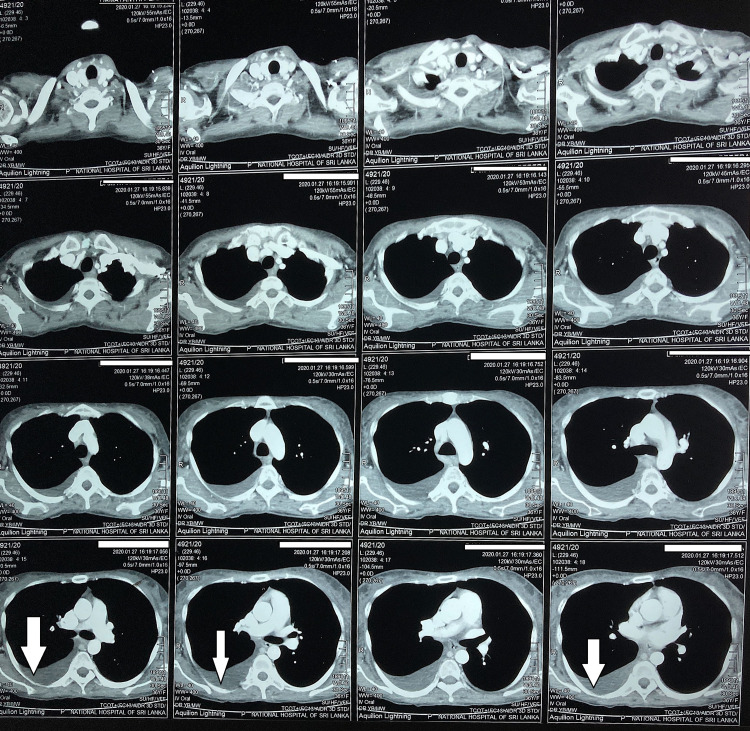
CT chest, abdomen and pelvis show right-sided pleural effusion.
